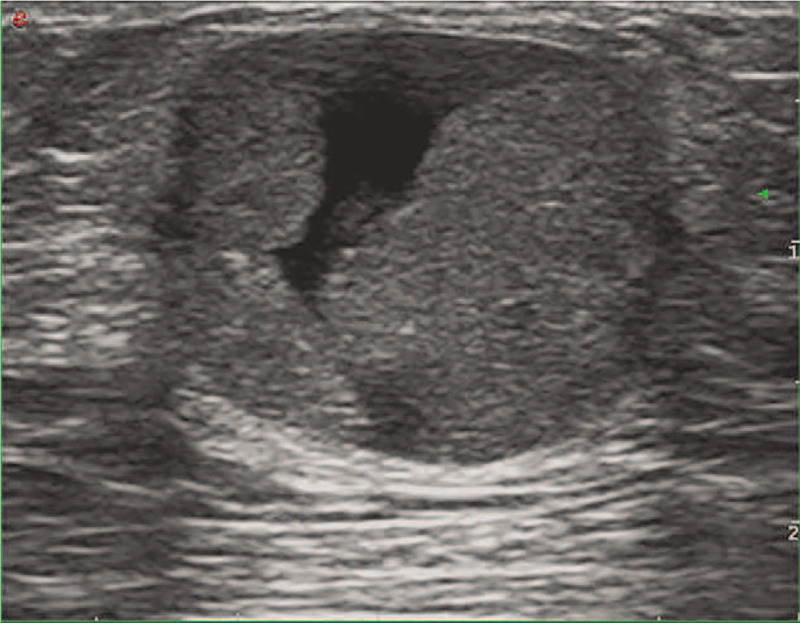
The long-axis sonogram of the left breast showed a regular shaped, well-defined complex mass with the cystic and solid hypoechoic mass in the retro areolar region.
